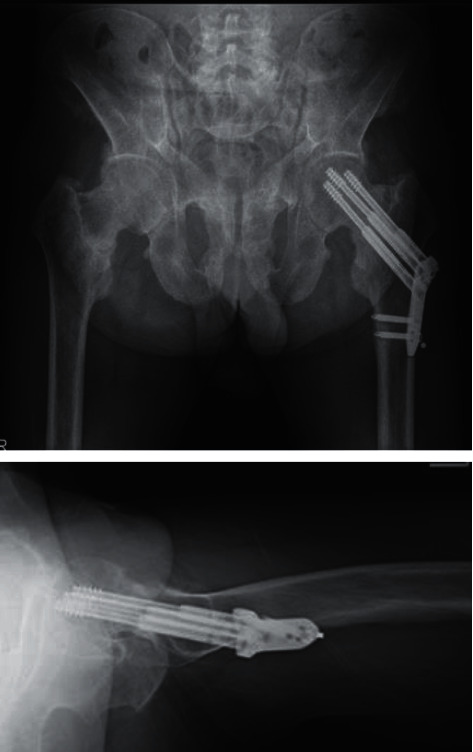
Radiographs taken 15 months after surgery revealing that the fracture achieved bone union.
